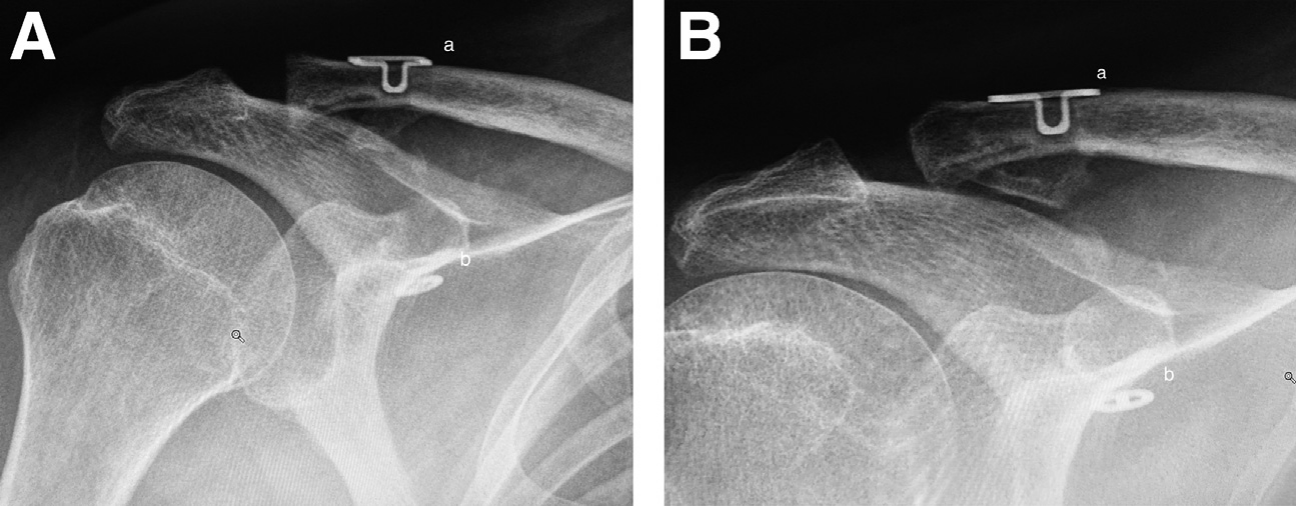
(A) A postoperative radiograph of the coracoclavicular CC reconstruction. The Clavicular Clip (a), the Subcoracoid Clip (b). Anteroposterior view, right shoulder. (B) A one-year postoperative radiograph of the CC reconstruction. The Clavicular Clip (a), the Subcoracoid Clip (b). Anteroposterior view, right shoulder.
